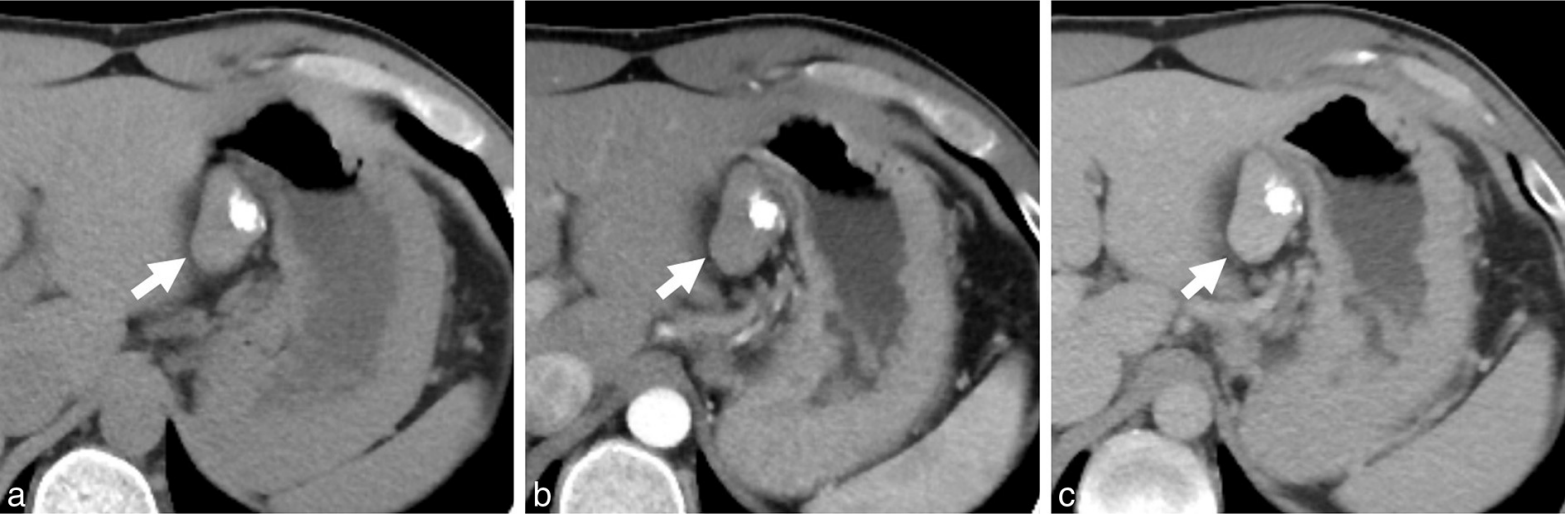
Findings on CT scan. A 36 × 24 mm mass is located in the lesser curvature of the stomach (arrows). The mass is calcified and slowly enhancing. (a) Plain image, (b) early phase in contrast dynamic study and (c) delayed phase.
